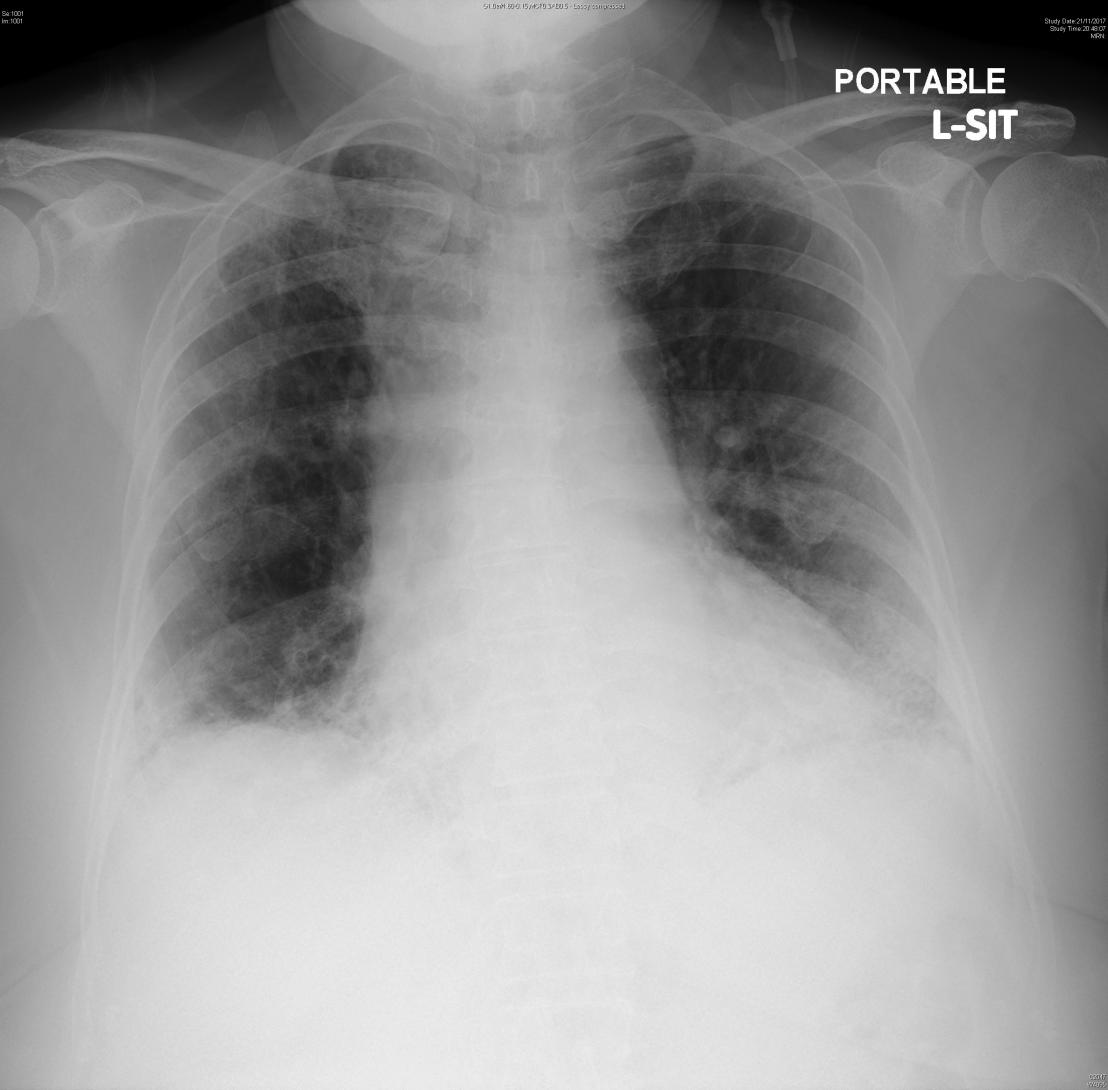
Chest radiograph on admission showing 1.3 cm right apical pneumothorax. Interstitial thickening, consistent with her history of idiopathic pulmonary fibrosis, is also evident especially in bilateral lower zones.
